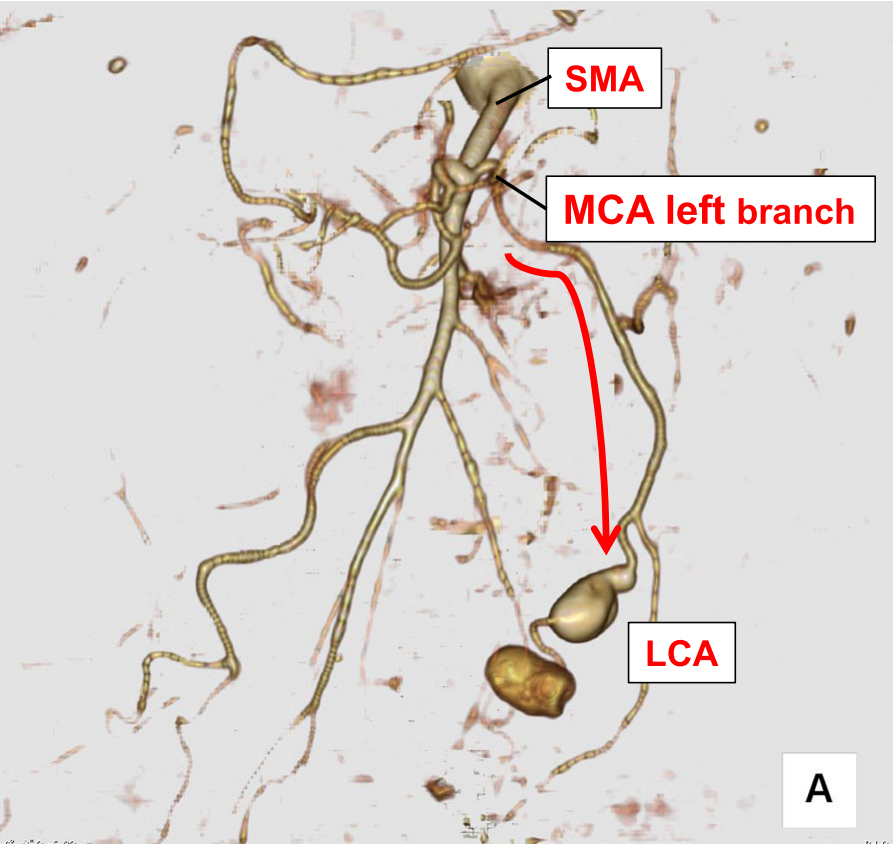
A 3D-CT angiography showing two sequential aneurysms in the left colic artery (LCA). The blood flow of the left colic artery was derived from the middle colic artery (MCA) left branch via the superior mesenteric artery (SMA) (red line)
